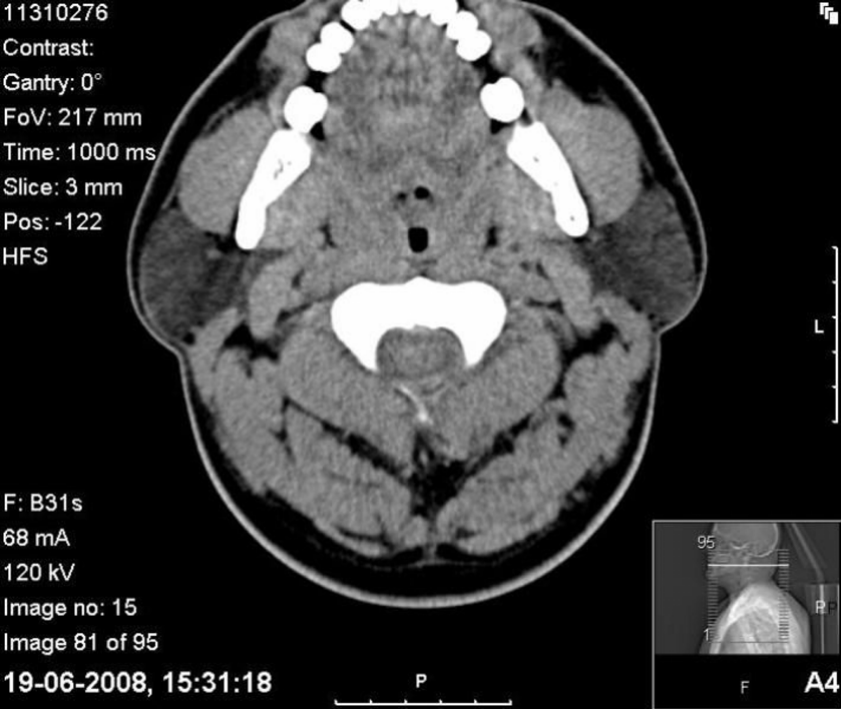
A facial computed tomography at 5.5 months following the initial presentation shows a smaller upper airway.
